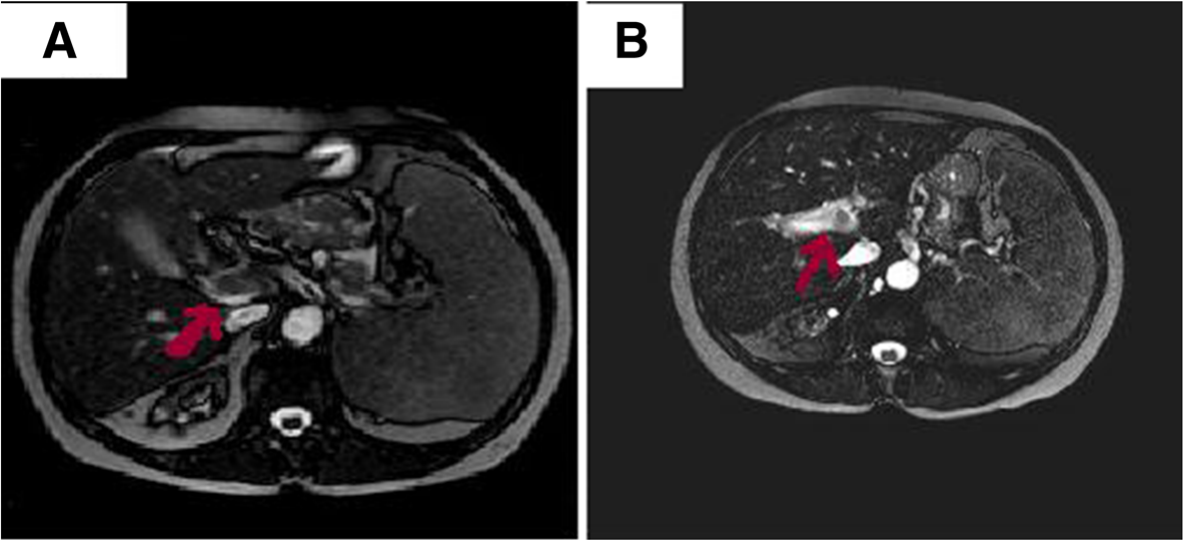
A: MR imaging of portal vein thrombosis before anticoagulant treatment. B: MRI of partial resolution of PV thrombus after warfarin treatment.
